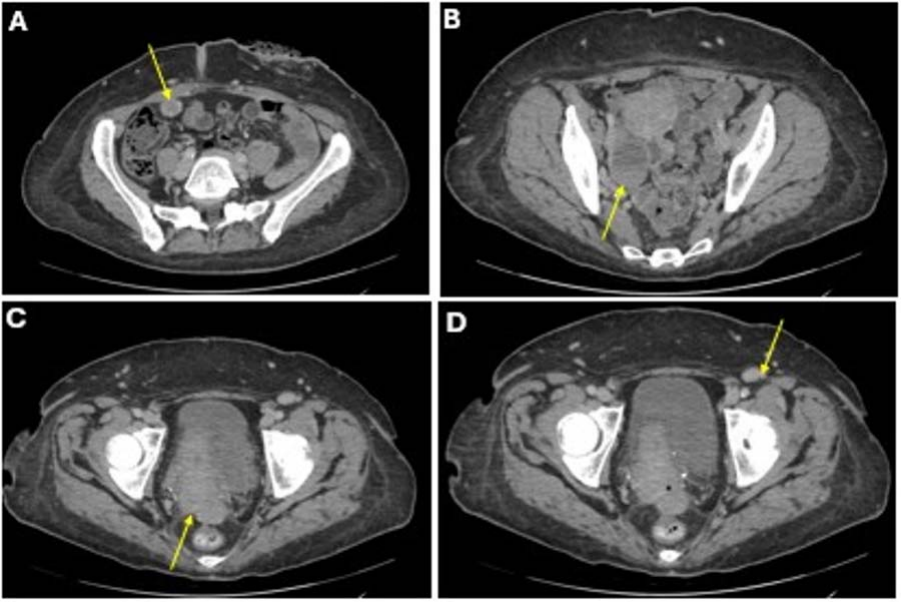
A CT imaging showing lesions involving the right abdominal wall (A), right ovary (B), right anterior reflection of the rectum (C), and left inguinal lymph node (D).
